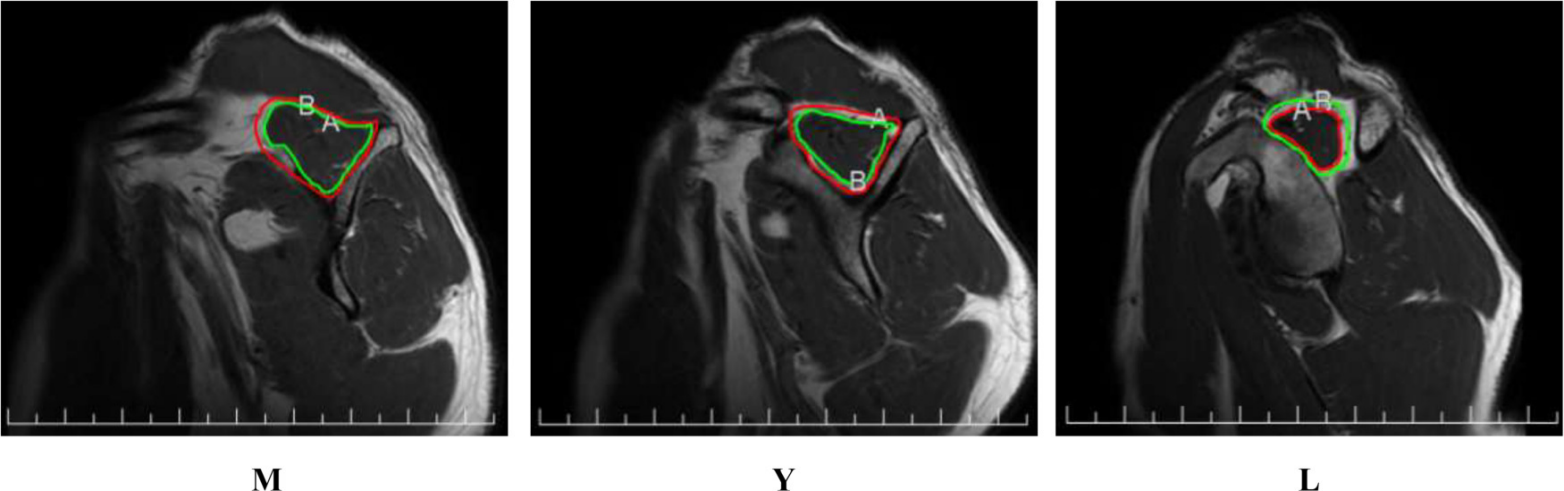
Three-slice levels in a shoulder magnetic resonance imaging oblique sagittal view. (M) The medial mid-slice (Y) The scapular Y-view (L) The lateral mid-slice [In the figures, the areas A (the area within the green line) and B (the area within the red line) were delineated using the PACS system. “A” denotes the residual muscle area, while “B” represents the total area of the supraspinatus muscle.].
